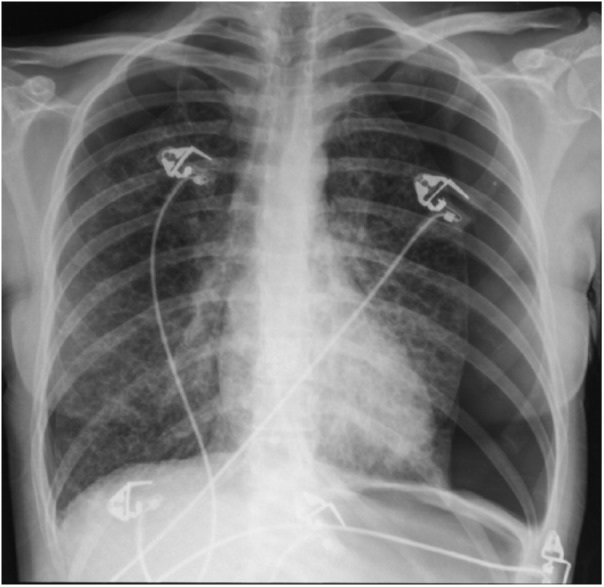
Chest radiograph with bilateral pneumothorax at presentation.
